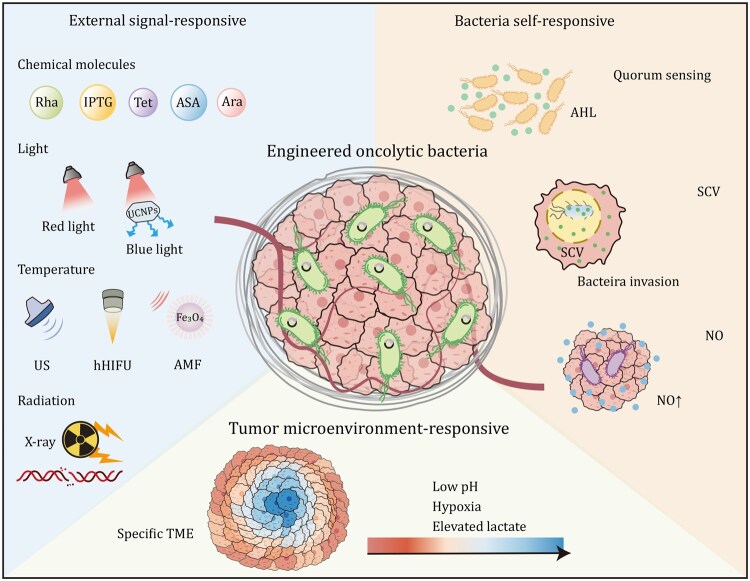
Controllable gene expression strategies for oncolytic bacteria-mediated precise tumor therapy. External signal-induced systems utilize chemical inducers (e.g., l-rhamnose (Rha), isopropyl β-d-1-thiogalactopyranoside (IPTG), tetracycline (Tet), acetyl salicylic acid (ASA), and l-arabinose (Ara)), light (red or blue light), temperature modulation via ultrasound (US) or high-intensity focused ultrasound (hHIFU), alternating magnetic field (AMF), and radiation (e.g., X-rays) to trigger gene expression. Bacterial self-responsive systems exploit intrinsic bacterial processes, such as quorum sensing via N-acyl homoserine lactones (AHL) or nitric oxide (NO) production by bacteria as triggers. Additionally, *Salmonella*-containing vacuoles (SCVs) sense the intravacuolar environment after bacterial invasion, activating SPI-2 promoters for tumor-specific payload release. Tumor microenvironment (TME) features, including hypoxia, low pH, elevated lactate levels, and nutrient abundance, also serve as inducers for precise therapeutic gene expression.
